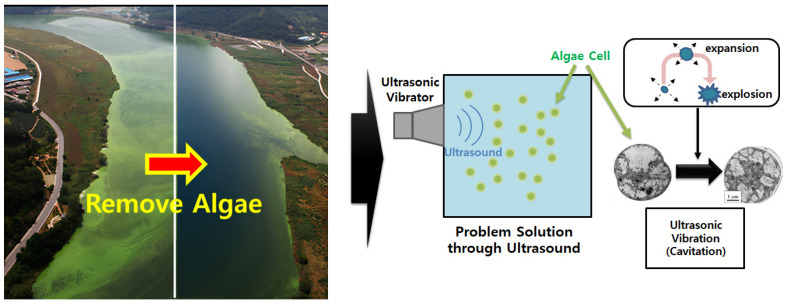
Environmental problems caused by algae and air sac destruction in cells via ultrasonic action.
